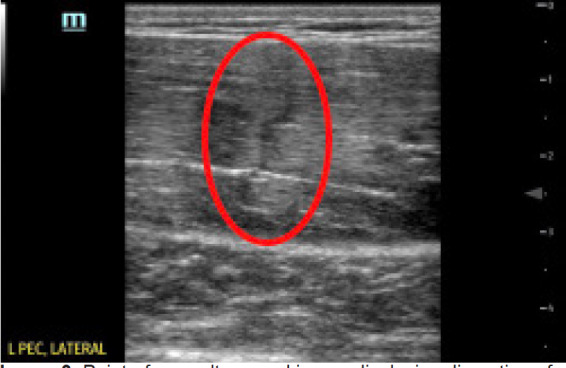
Point-of-care ultrasound image displaying disruption of the distal pectoralis major muscle bellies with vertical interruption noted (red circle).
